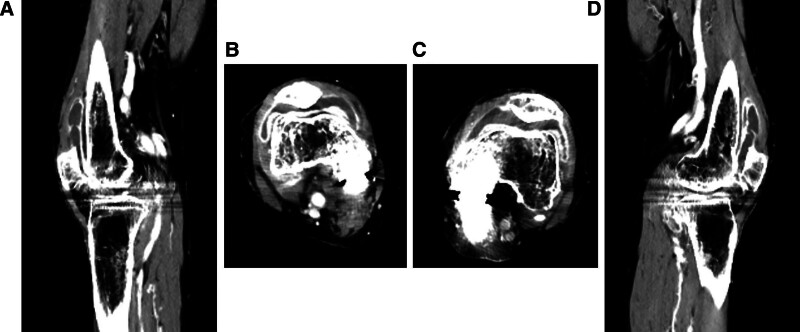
(A) The sagittal images of contrast-enhanced CT for the right knee joint. (B) The axial images of contrast-enhanced CT for the right knee joint. (C) The axial images of contrast-enhanced CT for the left knee joint. (D) The sagittal images of contrast-enhanced CT for the left knee joint.
